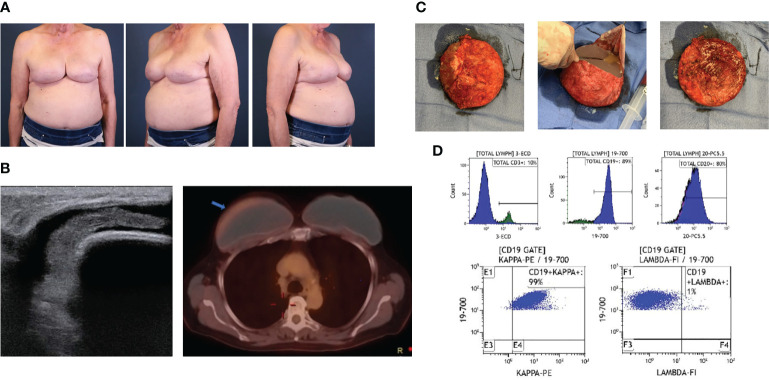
Case of BIA-DLBCL. (A) A 71-year-old female with history of left ductal carcinoma *in situ* status post bilateral mastectomies and immediate microtextured breast implant reconstruction in 2015 presented with unilateral right breast swelling three years after implantation with seroma aspirate consistent with BIA-DLBCL. (B) Ultrasound demonstrated peri-implant fluid collection and PET/CT revealed minimally increased metabolic uptake around the right breast capsule. (C) Bilateral *en bloc* capsulectomies were performed, demonstrating thick implant capsules and yellow-tinged intracapsular fluid collection. (D) Flow cytometry from the right breast capsule revealed a majority of cells expressing CD19 and CD20 with kappa light-chain restriction. No additional adjunct therapies were required. She has had a complete response and has no evidence of recurrence at 3 years post-operatively.
